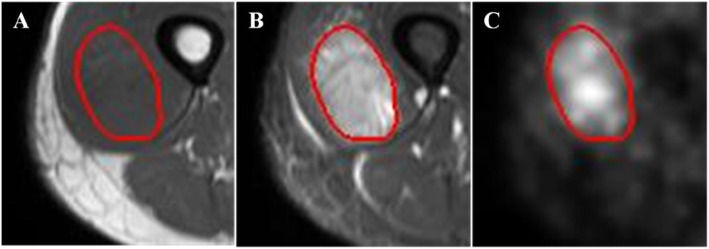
An example of tumor delineation (red line) in PET and MR images of a patient with STS. A T1-weighted MR images; B T2-weighted MR images; C PET images
